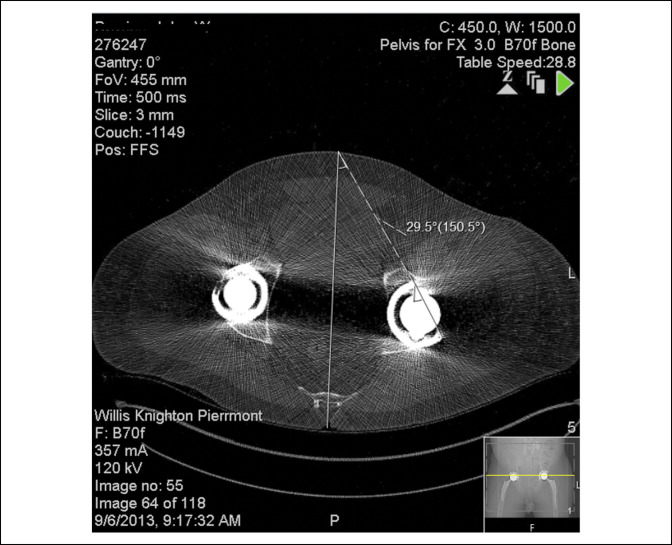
CT scan of the revision left total hip arthroplasty revealing that new cup position is 29.5° of anteversion.
